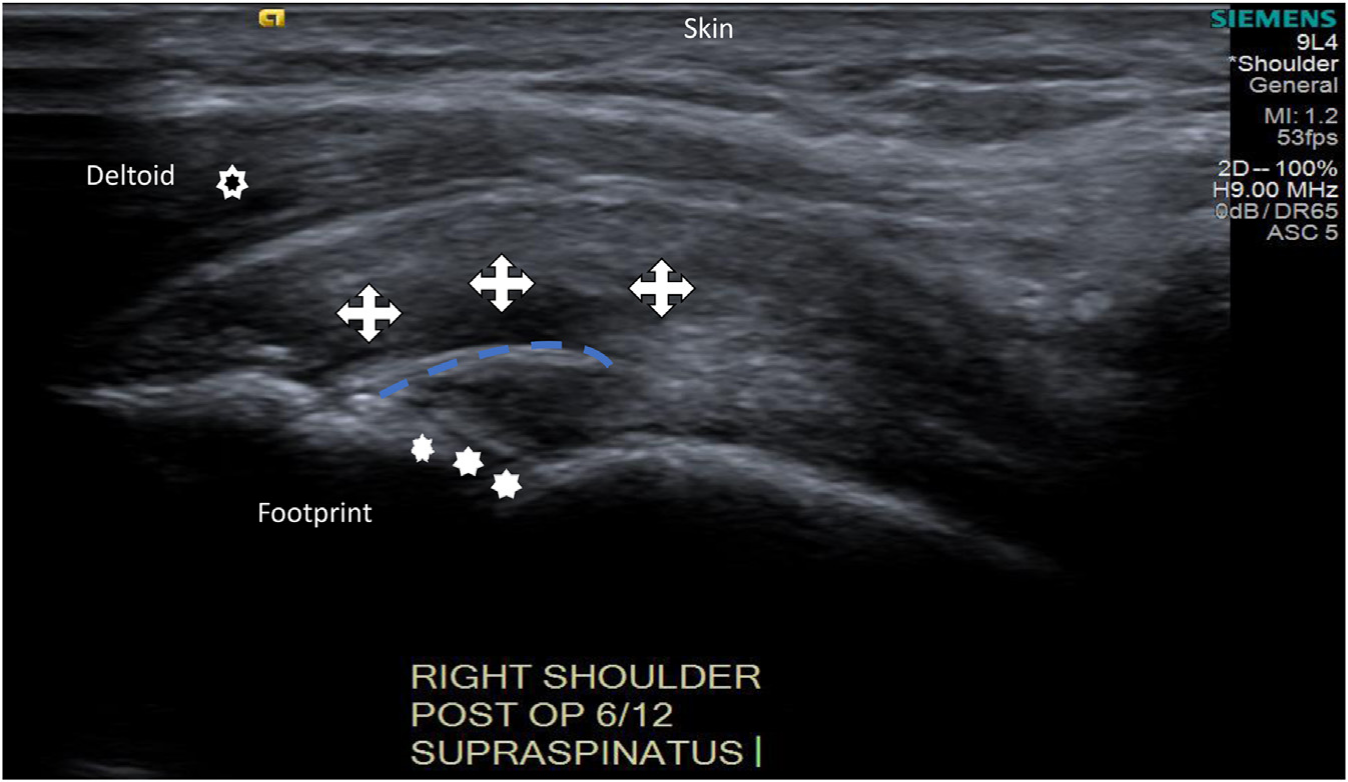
Ultrasound image showing three distinct supraspinatus tendon location (crosses), humeral head (stars), and deltoid (hollow circle) measurements 3 mm apart.
